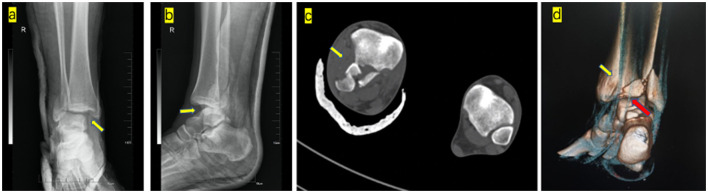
First attempted closed reduction of images: (a) Anteroposterior radiograph shows medial malleolus the axilla sign (yellow arrow). (b) Lateral radiograph show posterior subluxation of the talus. Axial CT scan (c) showing that the proximal fragment of the fibula was locked between the anterior part of the fibular notch and the posterior tibial fragment (yellow arrow). CT soft tissue reconstruction showing posterior tibial tendon (d) (yellow arrow) and flexor hallucis longus (d) (red arrow) located in the anatomical position.
